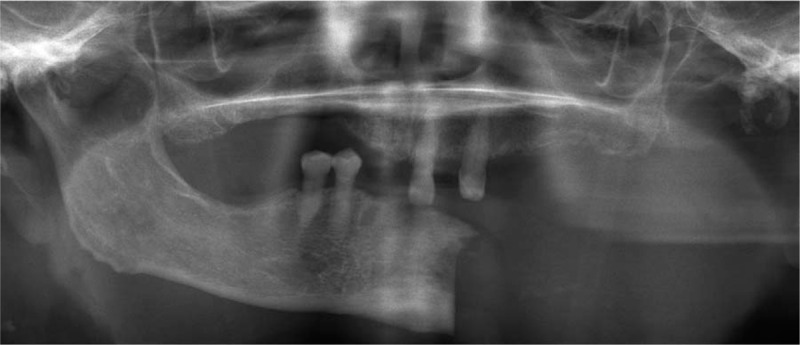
Follow-up orthopantomography 2 weeks after hemimandibulectomy.
